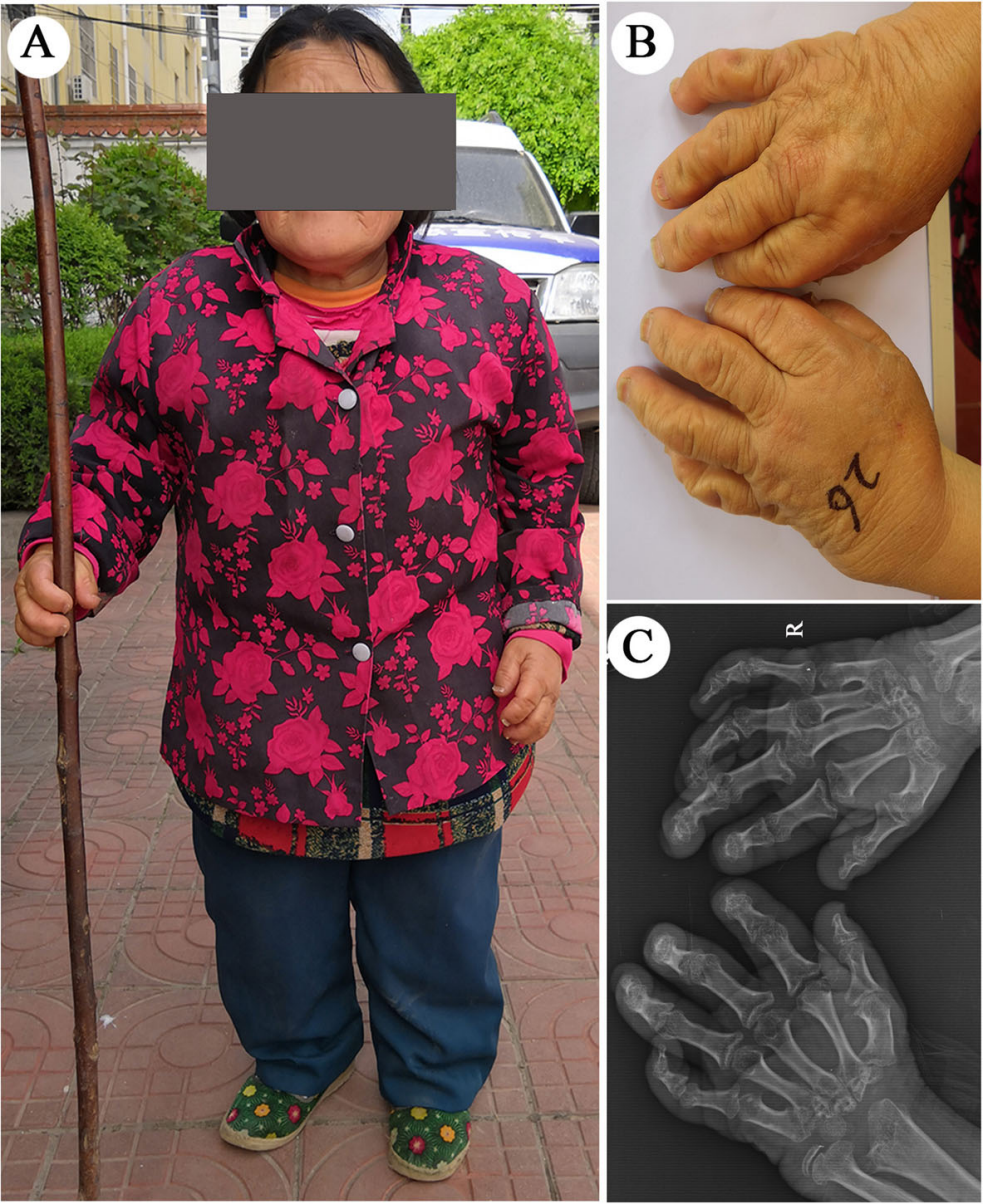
Characteristics of Kashin-Beck disease (KBD). a A representative patient severely affected with KBD (female, 57 years old, height 102 cm), which manifested as deformed and shortened fingers, a short stature and limited motion of the joints in the extremities. b & c Images and radiographic images of the bilateral hands of this patient, showing deformed fingers and metaphyseal lesions in the phalanges
